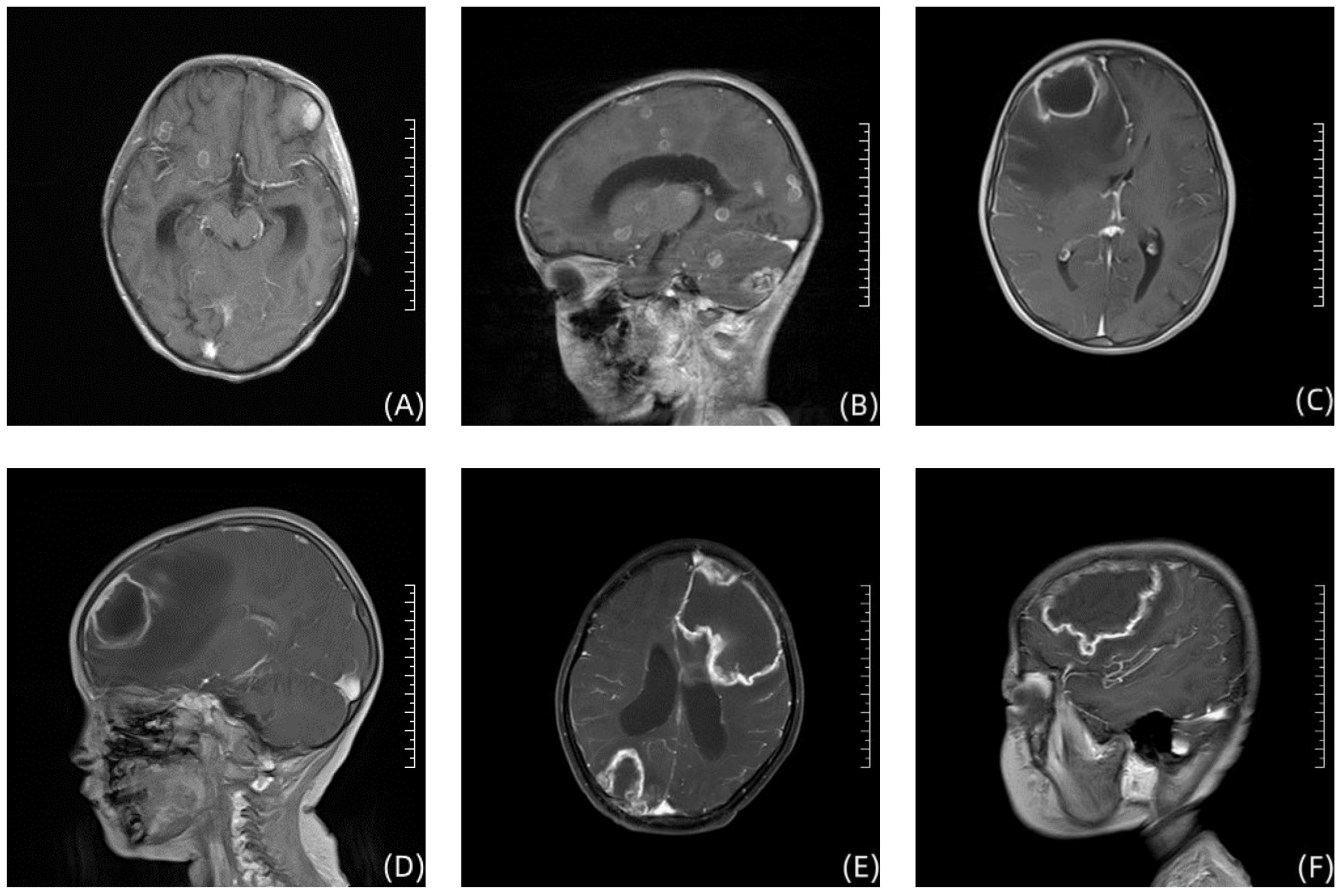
Enhanced T1-weighted brain MRI image in 3 children. (A, B) show the Brain imaging of Case 1; (C, D) show the Brain imaging of Case 2; (E, F) show the Brain imaging of Case 3.
